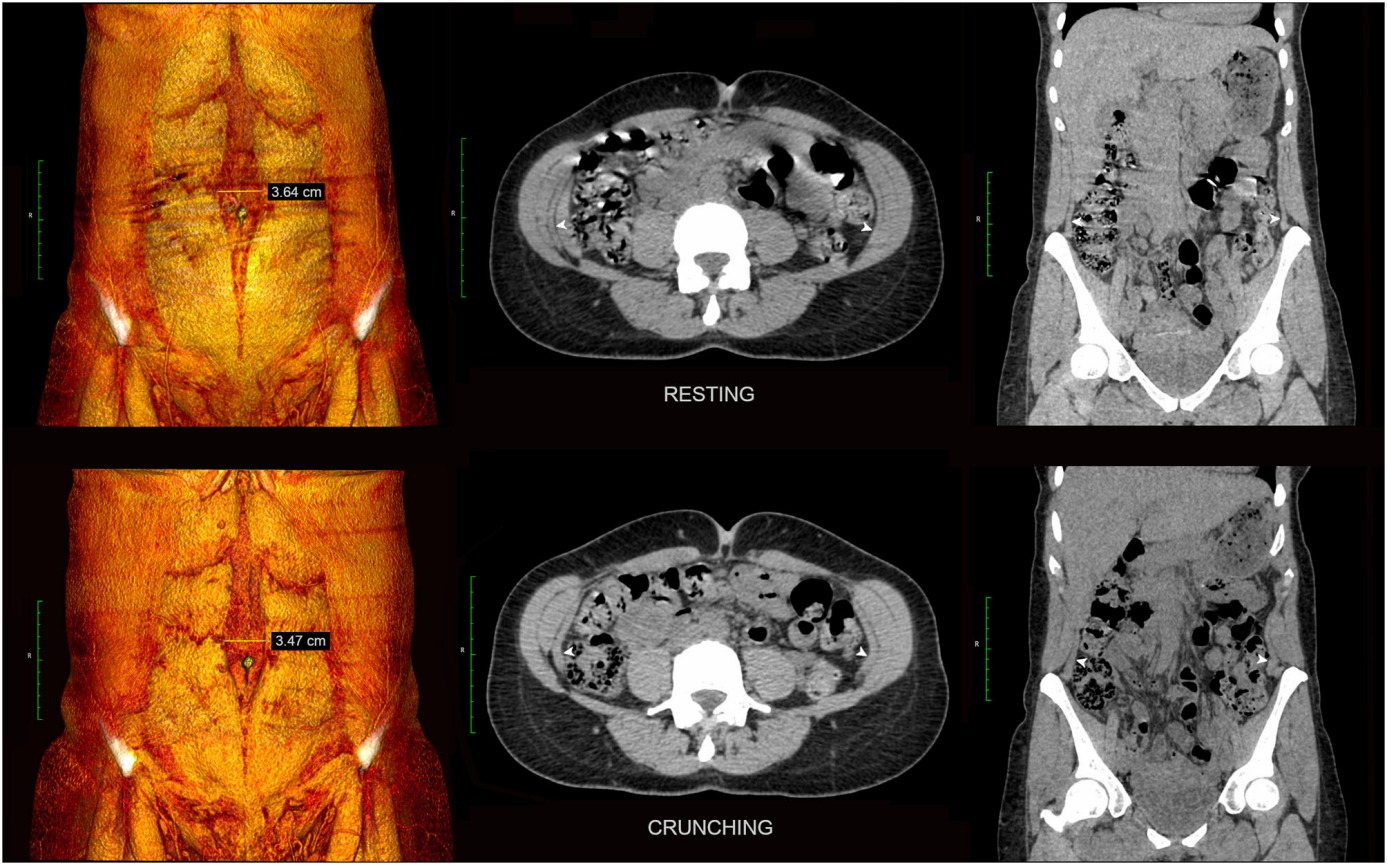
Diastasis rectus abdominis *without* dysfunctional bulge. Resting and crunching 3DVR (left) and axial/coronal 2D images (center and right) are shown from the functional CT examination of a 46 yearr old female with diastasis rectus abdominis. Note strong compensatory contractility of the entire EO + IO + TA muscle complex on each side (TA indicated by white arrowheads) sufficient in this case to maintain normal abdominal wall contour.
